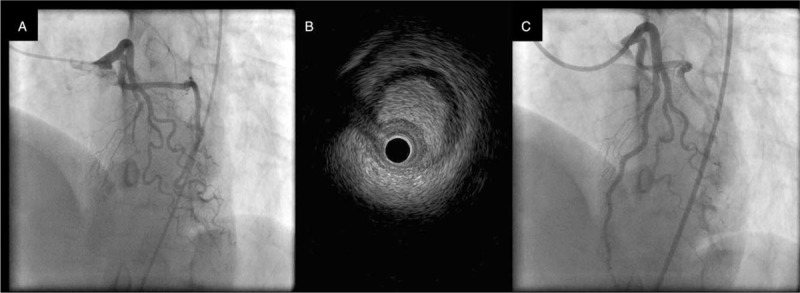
(A) Coronary angiography on the seventh day of hospitalization. The left anterior descending artery flow was TIMI grade 0. (B) We performed intravascular ultrasonography after balloon dilatation of the lesion. The true lumen was occluded by an intramural hematoma that developed in the false lumen. (C) We performed percutaneous coronary intervention, and the left anterior descending artery became TIMI grade 3.
